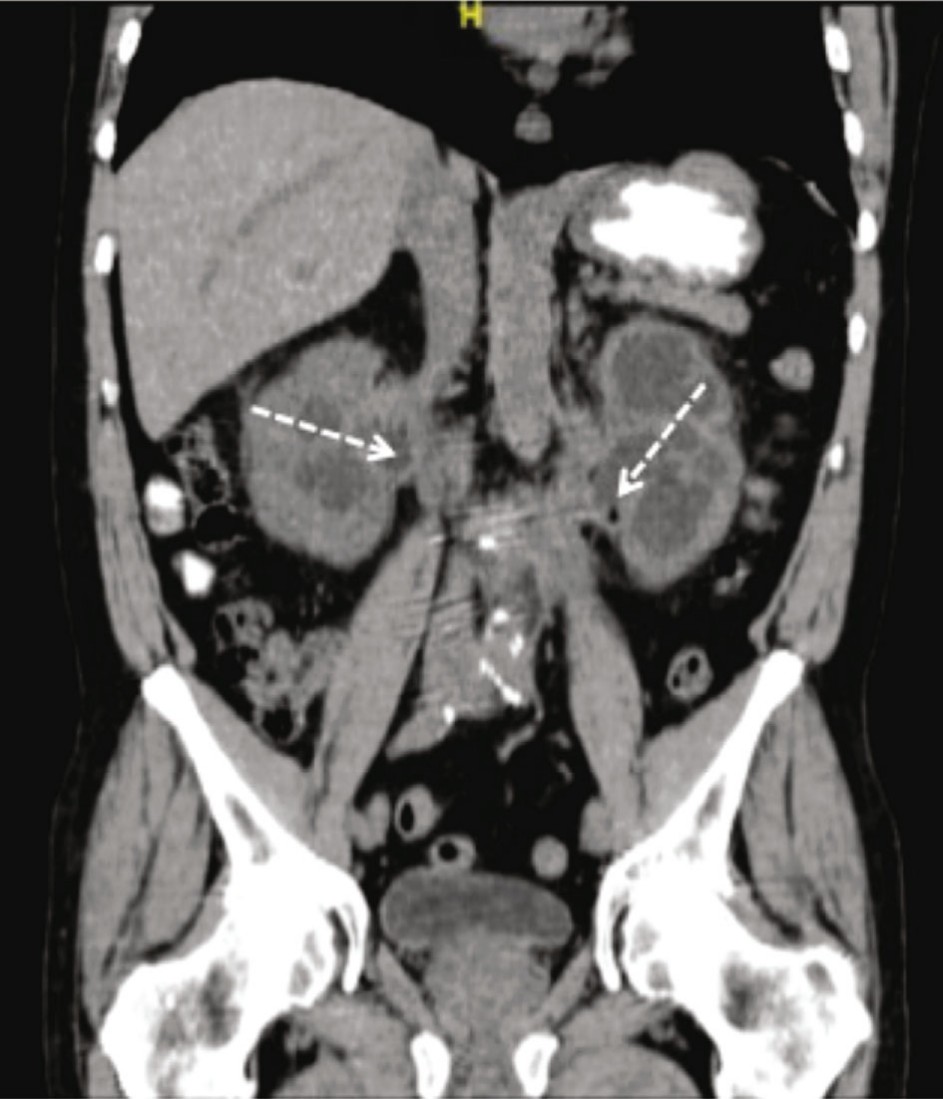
Computed tomography images of abdomen and pelvis of which dashed arrows indicated obstruction.
